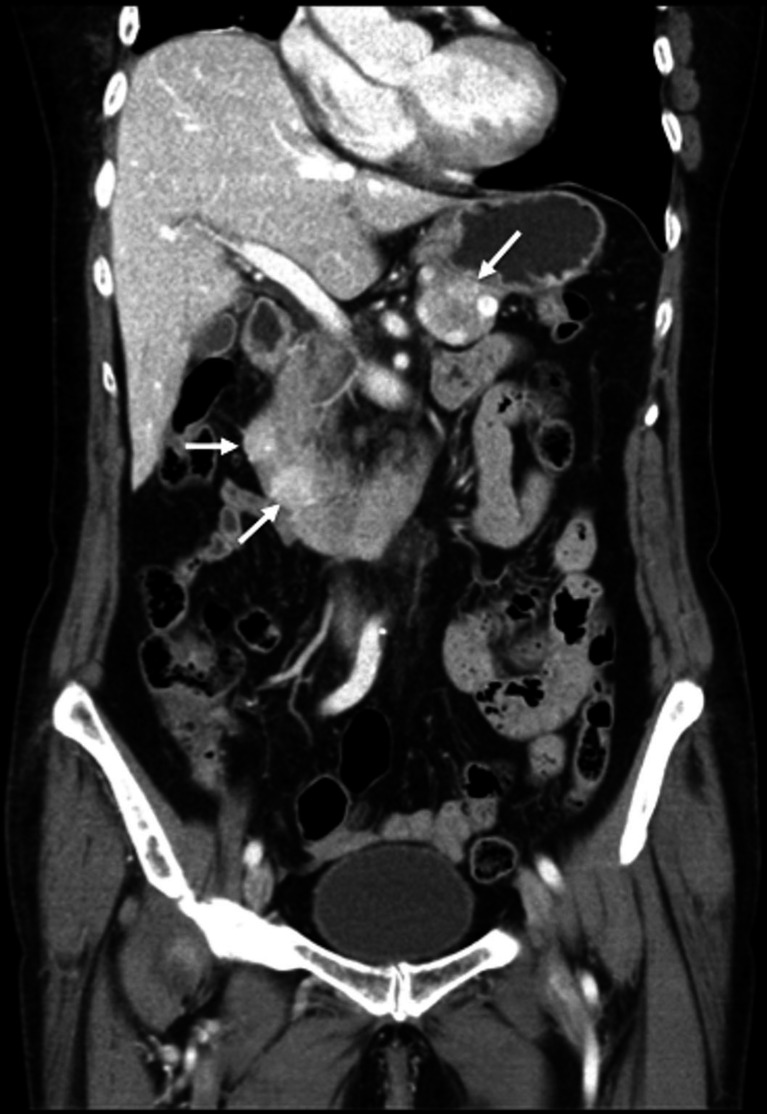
Coronal reformatted arterial phase contrast-enhanced multidetector CT image demonstrates multiple enteropancreatic neuroendocrine tumors (*arrows*) in a patient with MEN-1. These tumors and their metastases are often hypervascular. They are usually more conspicuous in the early arterial acquisition phase
